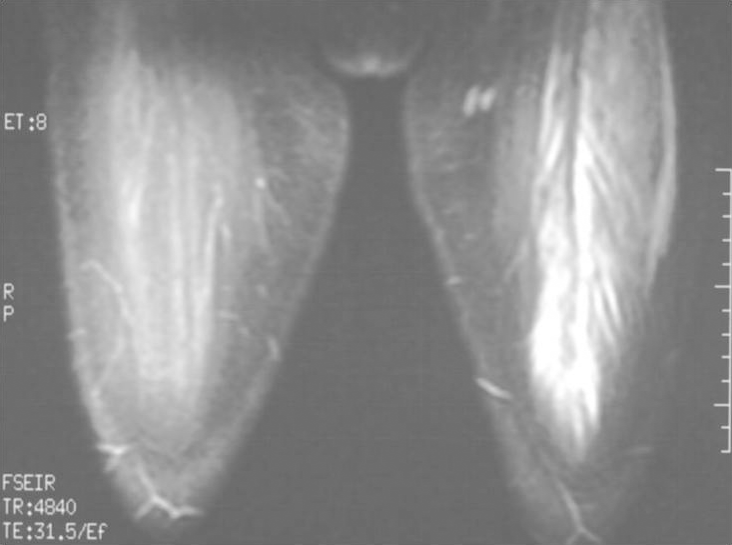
Coronal MRI section imaging haematoma of the left thigh.
